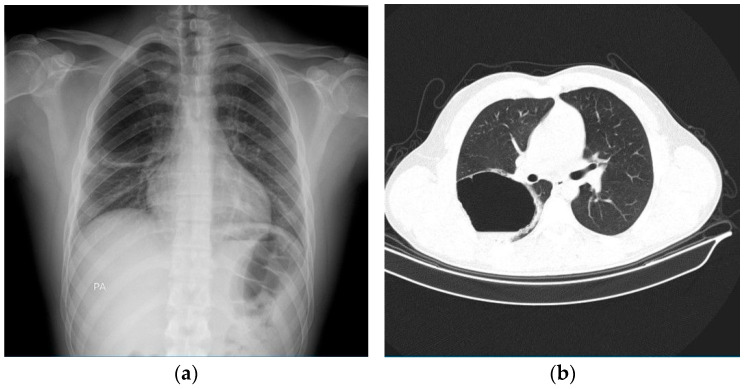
Imagistic investigations for diagnosis assessment upon admission. (a) Chest-X-ray shows hyper-transparent image with clear internal and external contours, homogeneous, oval, of 84/60 mm diameter (anteroposterior/craniocaudal), in the right middle lobe. (b) Lung CT scan shows a septate cystic lesion with air-fluid level (fluid component measuring 11 mm in thickness and air component measuring 66 mm), with a thin, irregular wall (approximately 3 mm thick), with overall dimensions of 77/84/62 mm (anteroposterior/transverse/craniocaudal), localized subpleural, in the posterior segment of the right upper lobe, with mass effect on the posterior half of the oblique fissure, apparently without communication with the bronchial tree.
